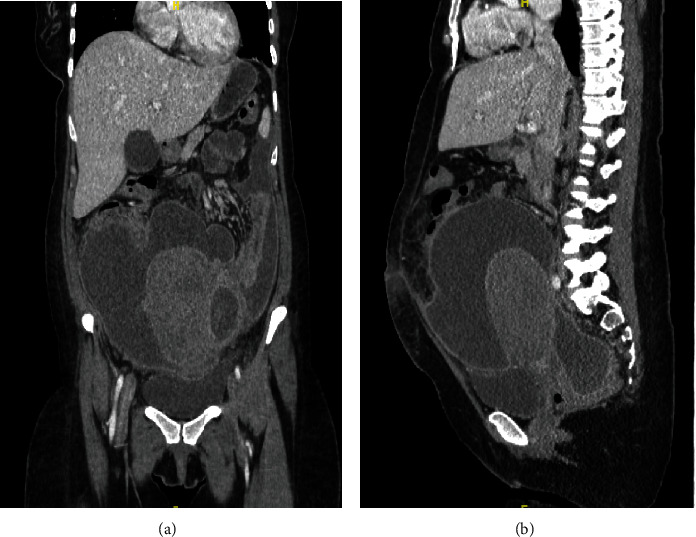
Coronal (a) and sagittal (b) views of the CT abdomen and pelvis with IV contrast performed in the emergency department demonstrating multiple, large loculated fluid-filled collections.
